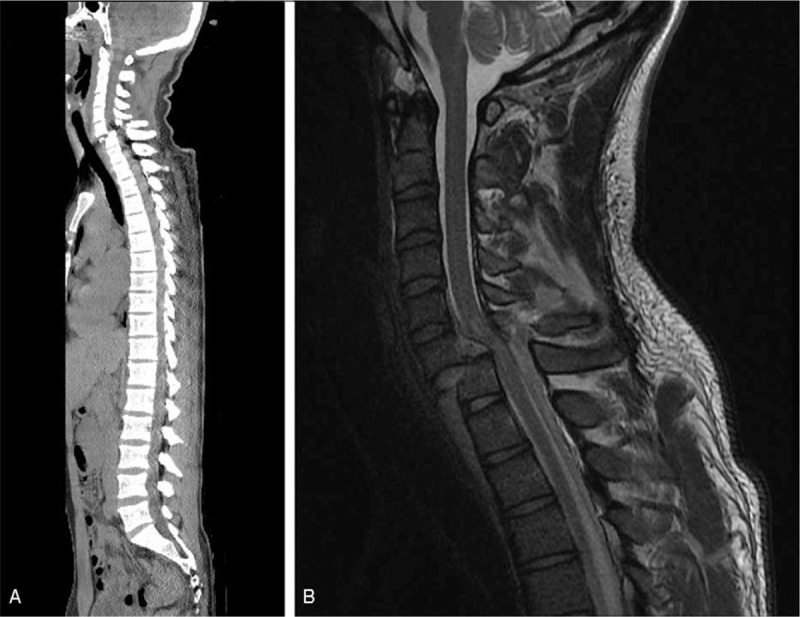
(A). Initial C-spine CT showing bilateral interfacetal dislocation on C6-7. (B) Initial C-spine MRI shows anterior translational injury at C6-7 with severe cord encroachment and complete discoligamentous complex disruption.
